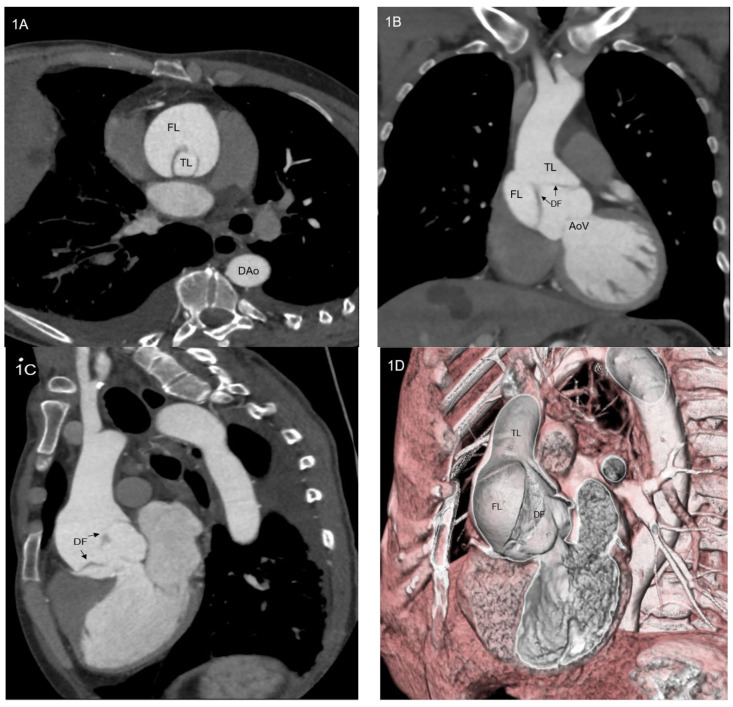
(A–D) Computed tomography angiogram of a patient with type A aortic dissection: axial scan (A), coronal scan (B), sagittal scan (C) and 3D volume rendering view. AoV = aortic valve; DAo = descending aorta; DF = dissection flap; FL = false lumen; TL = true lumen.
